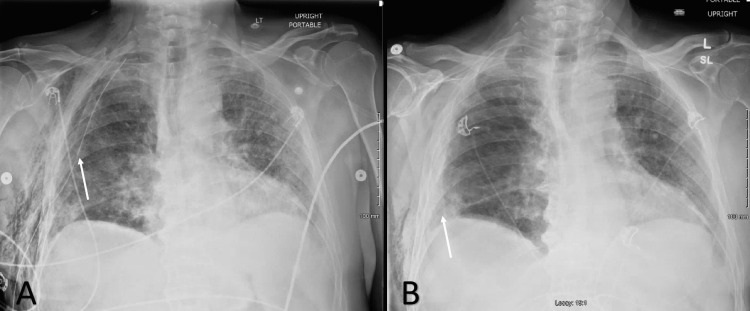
Anterior-Posterior chest x-ray A) Anterior-Posterior chest x-ray on postoperative day one showing no residual pneumothorax and improving atelectasis in the left lung spaces and right lung space with a right-sided chest tube, shown with a white arrow. B). Anterior-Posterior chest x-ray on the day of discharge twenty four hours after the patient’s right-sided chest tube was removed with minor residual lower lung lobe atelectasis indicated with a white arrow.
